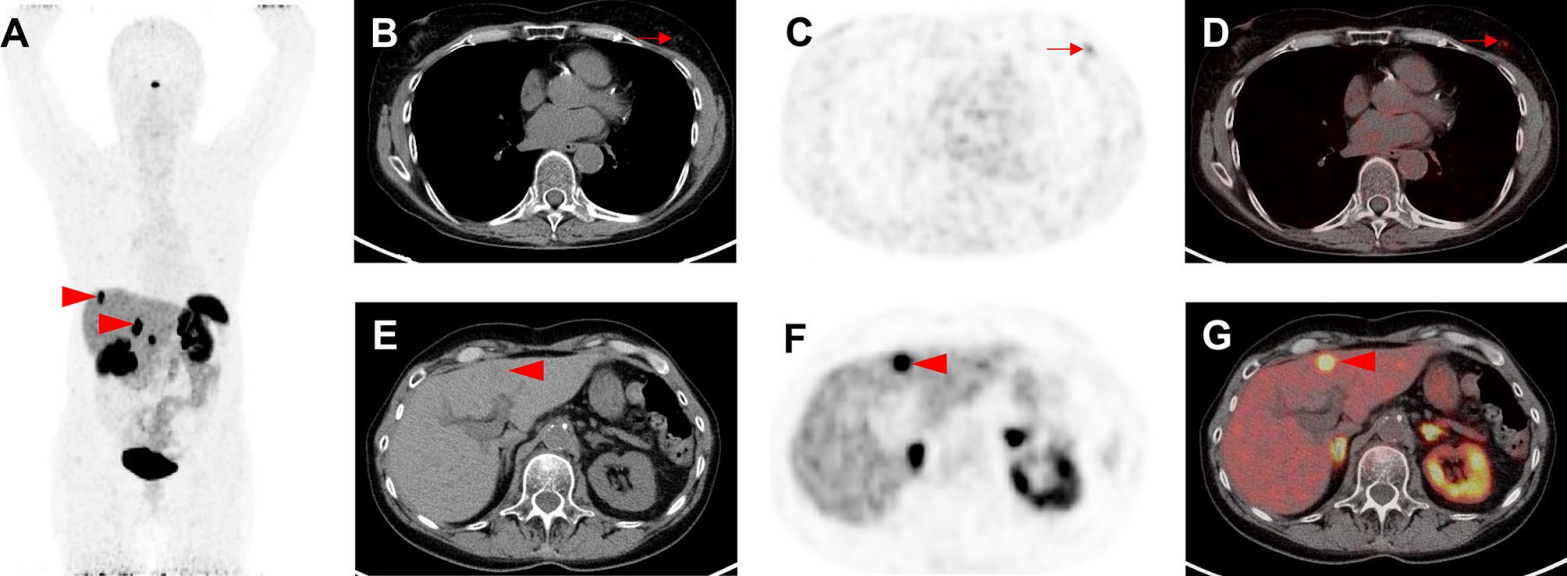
Indeterminate findings on somatostatin receptor (SSTR) positron emission tomography (PET)/computed tomography (CT). A 63-year-old man with history of a gastroenteropancreatic neuroendocrine tumor, who underwent [^68^Ga]-labeled 1,4,7,10-tetraazacyclododecane-*N,N*′,*N*′′,*N*′′′-tetraacetic acid-d-Phe(1)-Tyr(3)-octreotide ([^68^Ga]-DOTATOC) PET/CT for staging. a Whole-body maximum intensity projection image demonstrates suspicious radiotracer uptake (red arrowheads) in the liver. b Axial CT, c axial PET and d axial PET/CT show mild radiotracer uptake in the left breast (red arrows). This has been classified as SSTR-RADS-3C by an experienced reader. e Axial CT, f axial PET, and g axial PET/CT demonstrate intense radiotracer uptake in multiple liver lesions (one of which is demarcated by the red arrows). As there is subtle hypoattenuation in the liver on e axial CT, this finding was classified as SSTR-RADS-5 and, therefore, the overall SSTR-RADS score was 5. Based on this scoring, peptide receptor radionuclide therapy should be considered [20]
